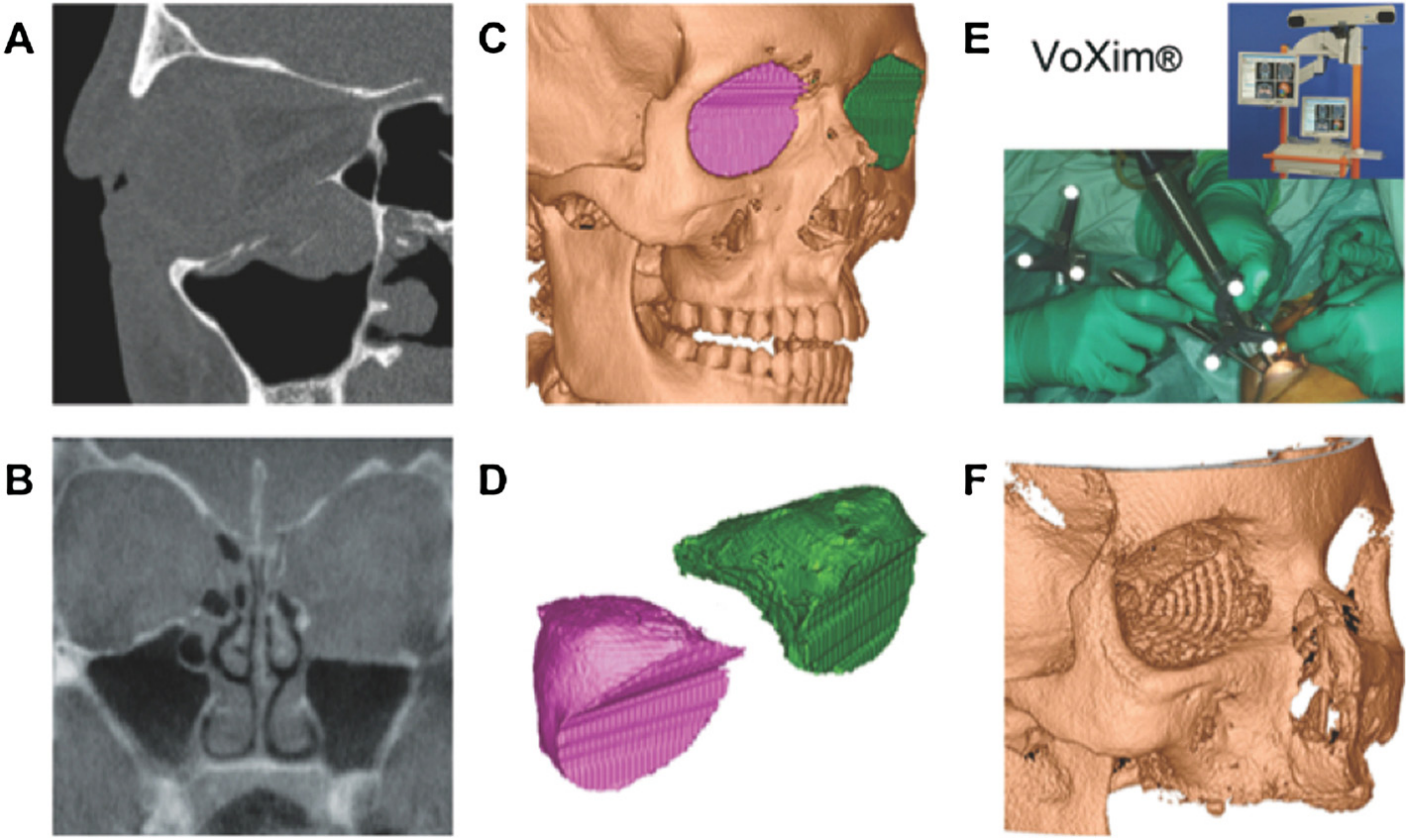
Image analysis in sagittal view (A) and coronal view (B), (C) pre-operative segmentation (CT scan), (D) both virtual segments, (E) intra-operative Navigation-assisted surgery using VoXim®, (F) post-operative control (CBCT-scan).
